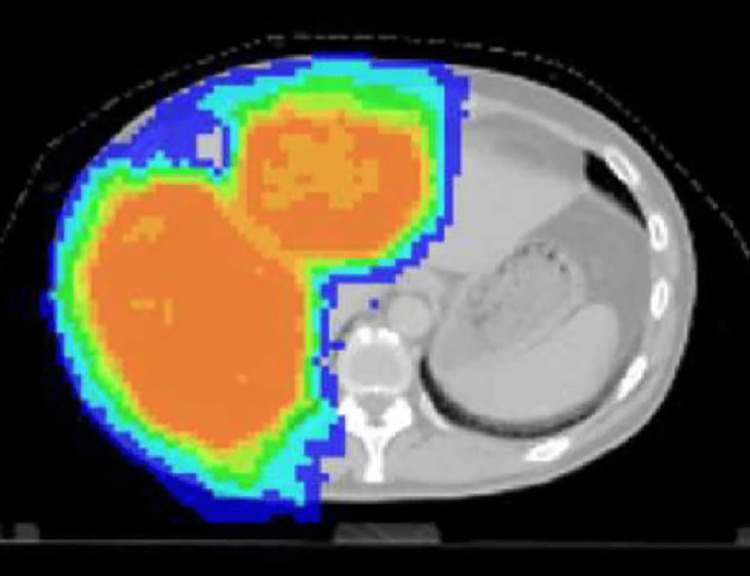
CT of the irradiated area before the start of WT1-DC The orange area is the main irradiated area. Cervical cancer metastases occupied 60% of the liver.
